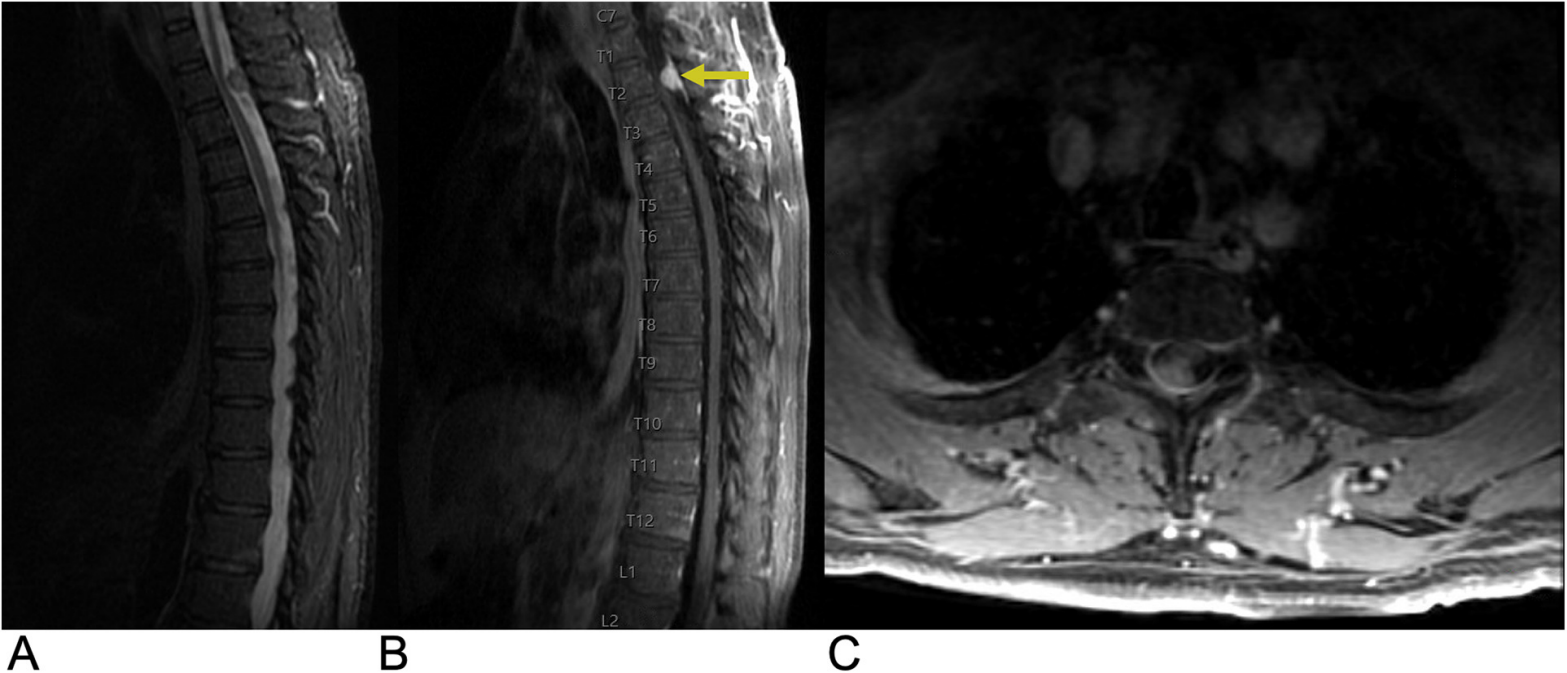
Meningioma. Sagittal T2 STIR (A), sagittal T1 FLAIR FAT + C (B), axial T1 FAT + C (C) MRI of the thoracic spine demonstrating an intradural, extramedullary oval-shaped enhancing lesion (yellow arrow) representing a spinal canal meningioma involving the right dorsolateral aspect of the thecal sac at the T2 level with moderate narrowing of the spinal canal.
